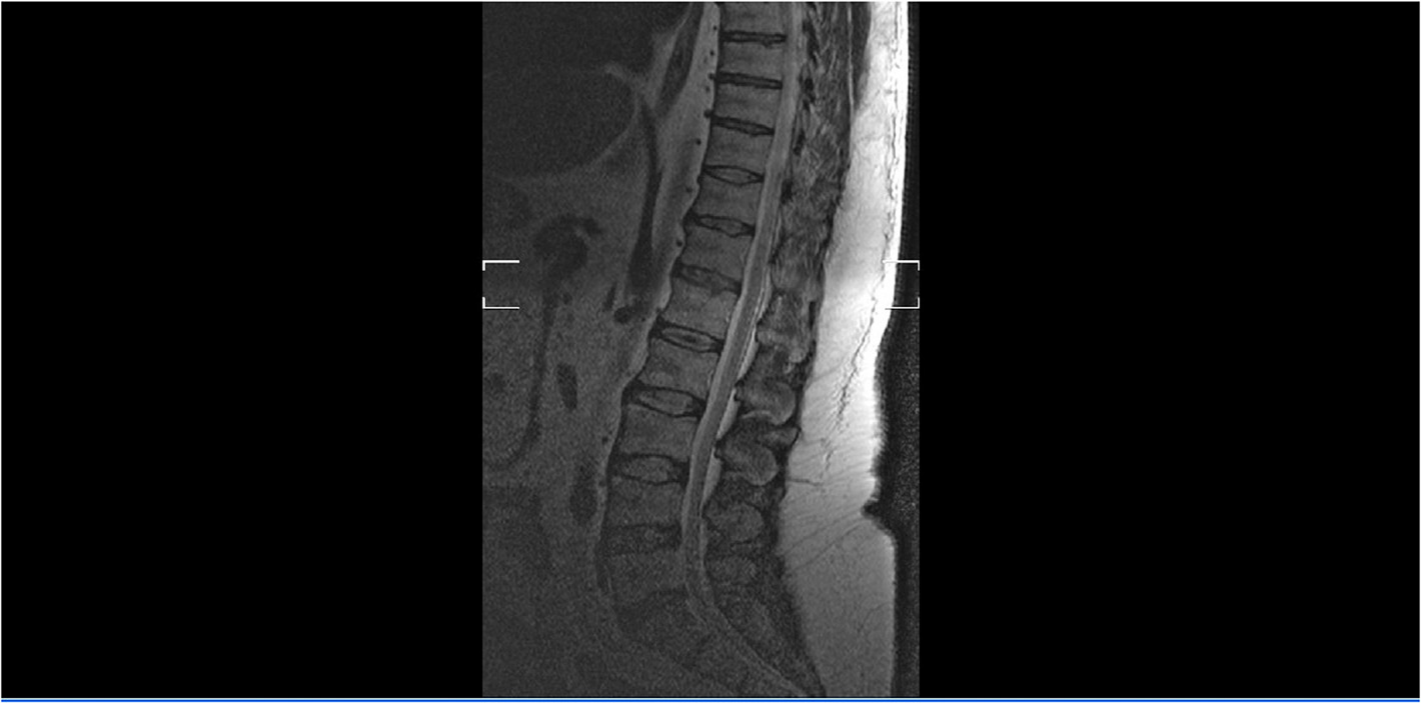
This lateral lumbar Magnetic Resonance Image demonstrates severe degenerative disc disease with a few bulging intervertebral discs
